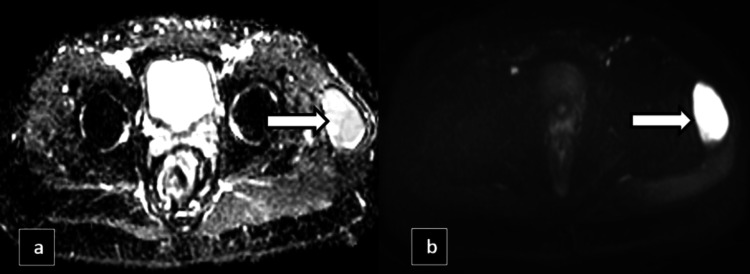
MRI axial reformatted apparent diffusion coefficient (ADC) image and MRI axial reformatted diffusion-weighted imaging (DWI) image (b) showing no true diffusion restriction (hyperintense signals on DWI and ADC) represented by the white arrows.
